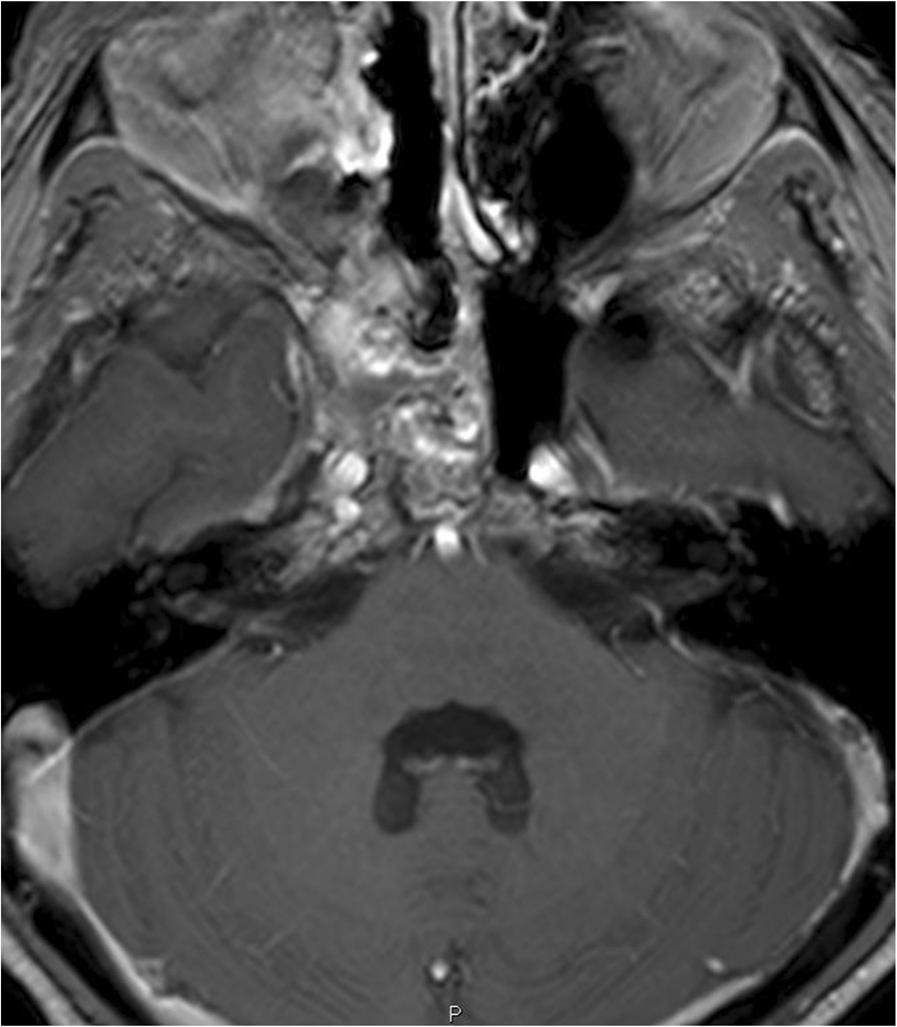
T1-weighted gadolinium enhanced images show a hypointense lesion of the clivus more towards the right side with destruction of the bony margins of the clivus. The lesion is found just anterior to the basilar artery. There was no contrast enhancement of the lesion.
